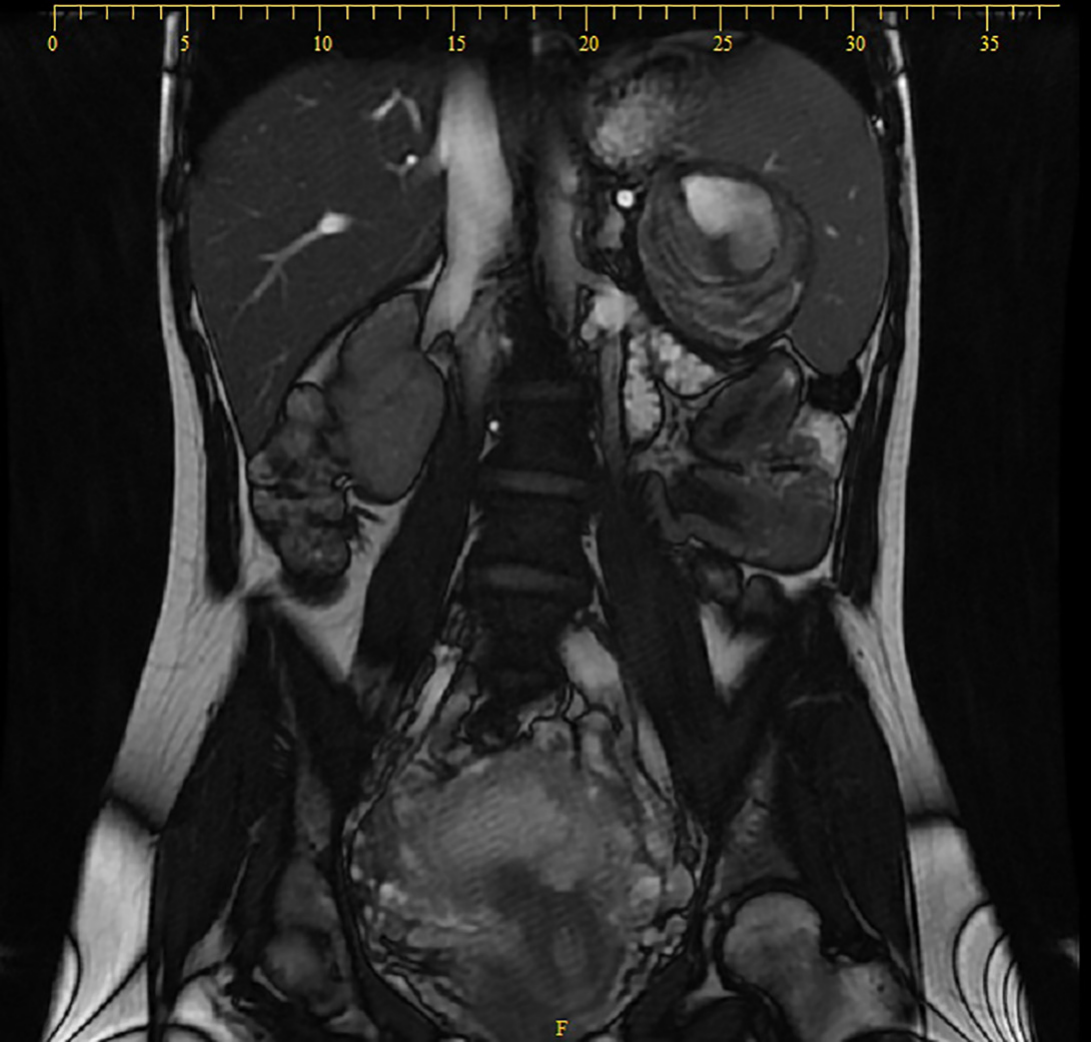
Coronal cut on MRI showing a 7.5 × 7 × 6.5 cm aneurysm in the distal portion of the splenic artery with an intramural thrombus, abutting the splenic hilum.
